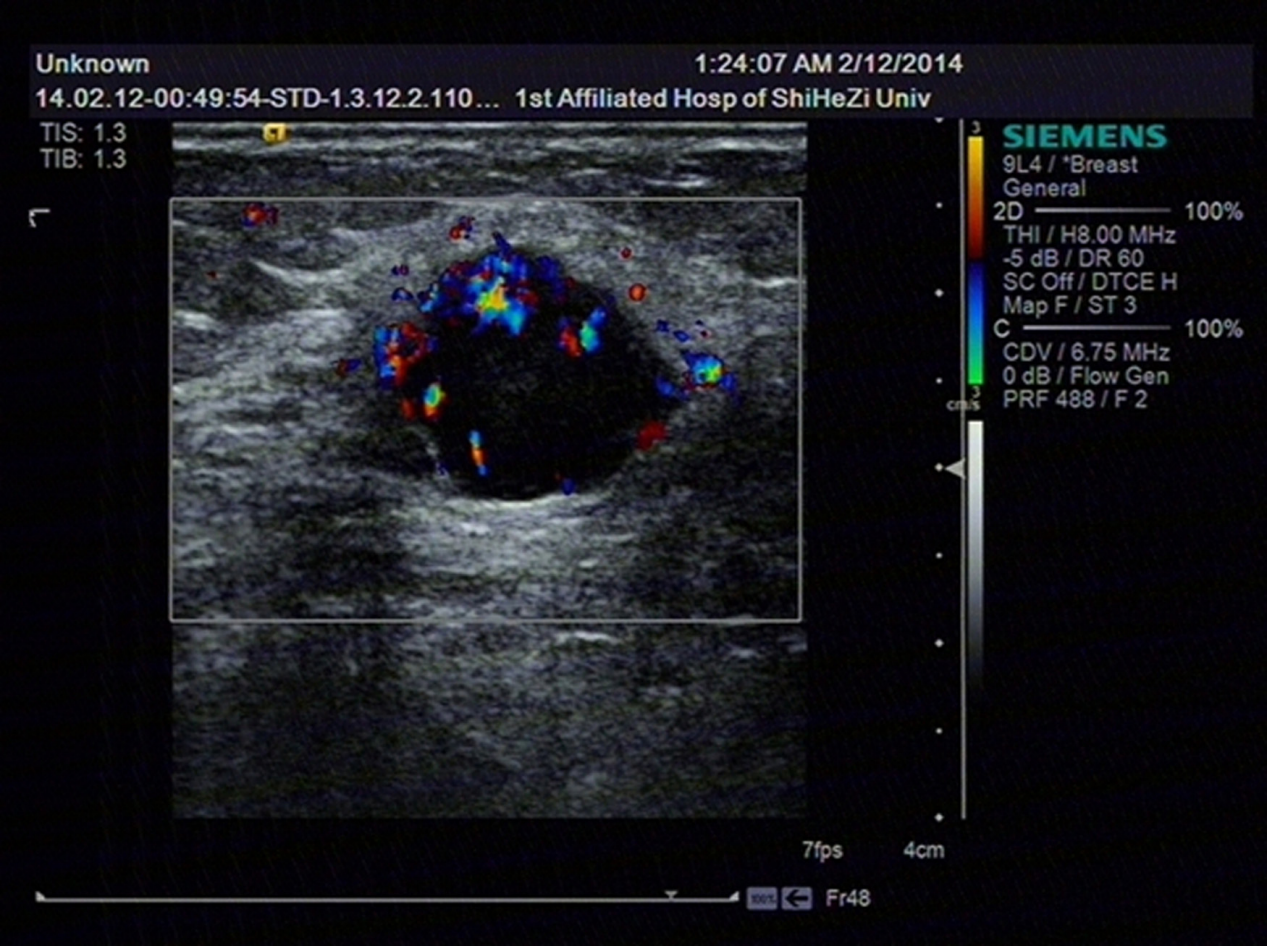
The left breast mass is shown in ultrasound as a low echo with a size of 2.4 cm × 1.5 cm.
